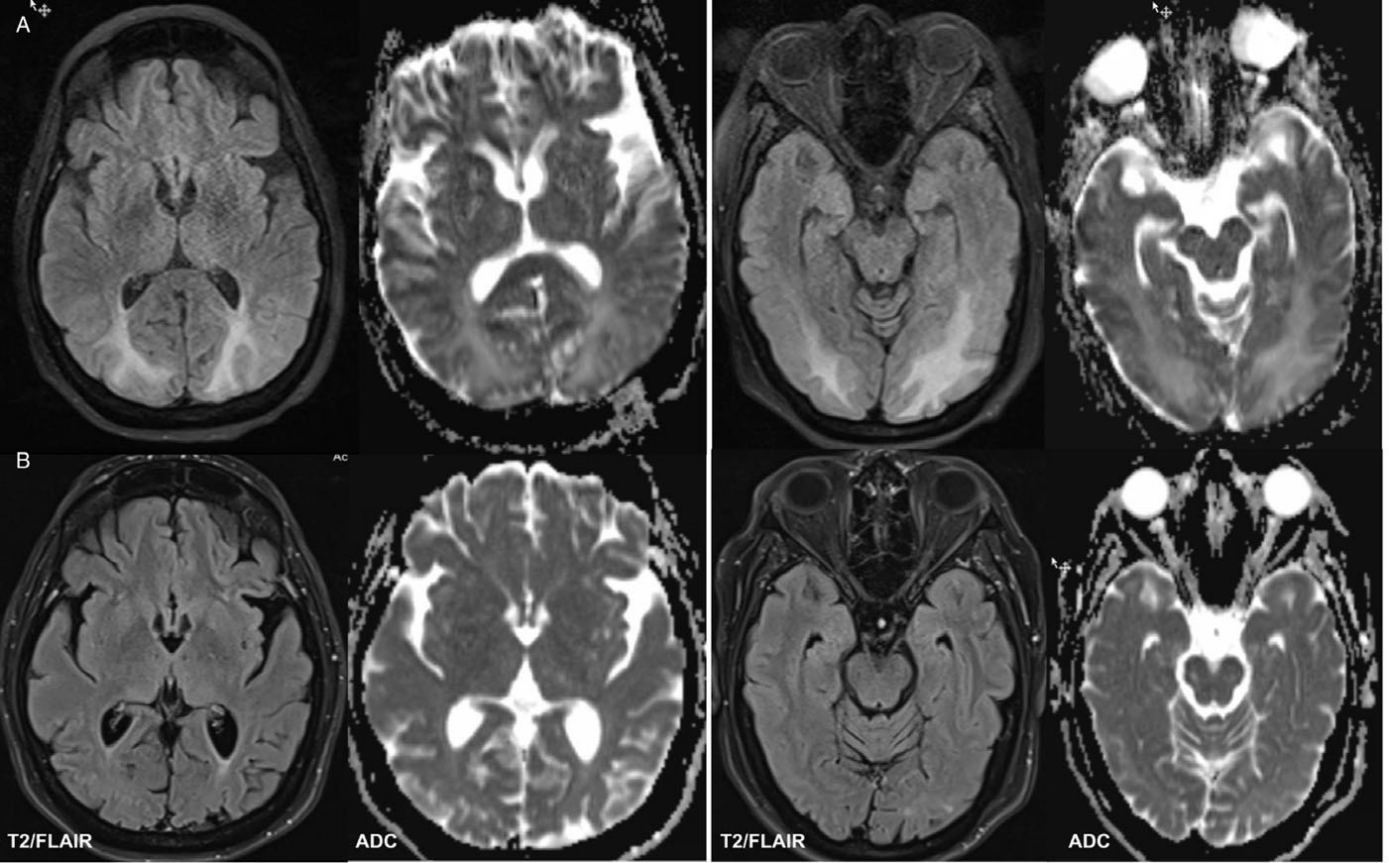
Posterior reversible encephalopathy syndrome in COVID-19. (A) Axial MRI images showing extensive bilateral regions of increased signal on T2/FLAIR sequences (left panels parieto-occipital, right panels occipital) and high signal on apparent diffusion coefficient sequences (ADC) consistent with extensive vasogenic edema. Most regions showed T2 shine through on diffusion-weighted imaging with sparse patches of restricted diffusion. (B) Follow-up MRI images showing resolution of edema.
